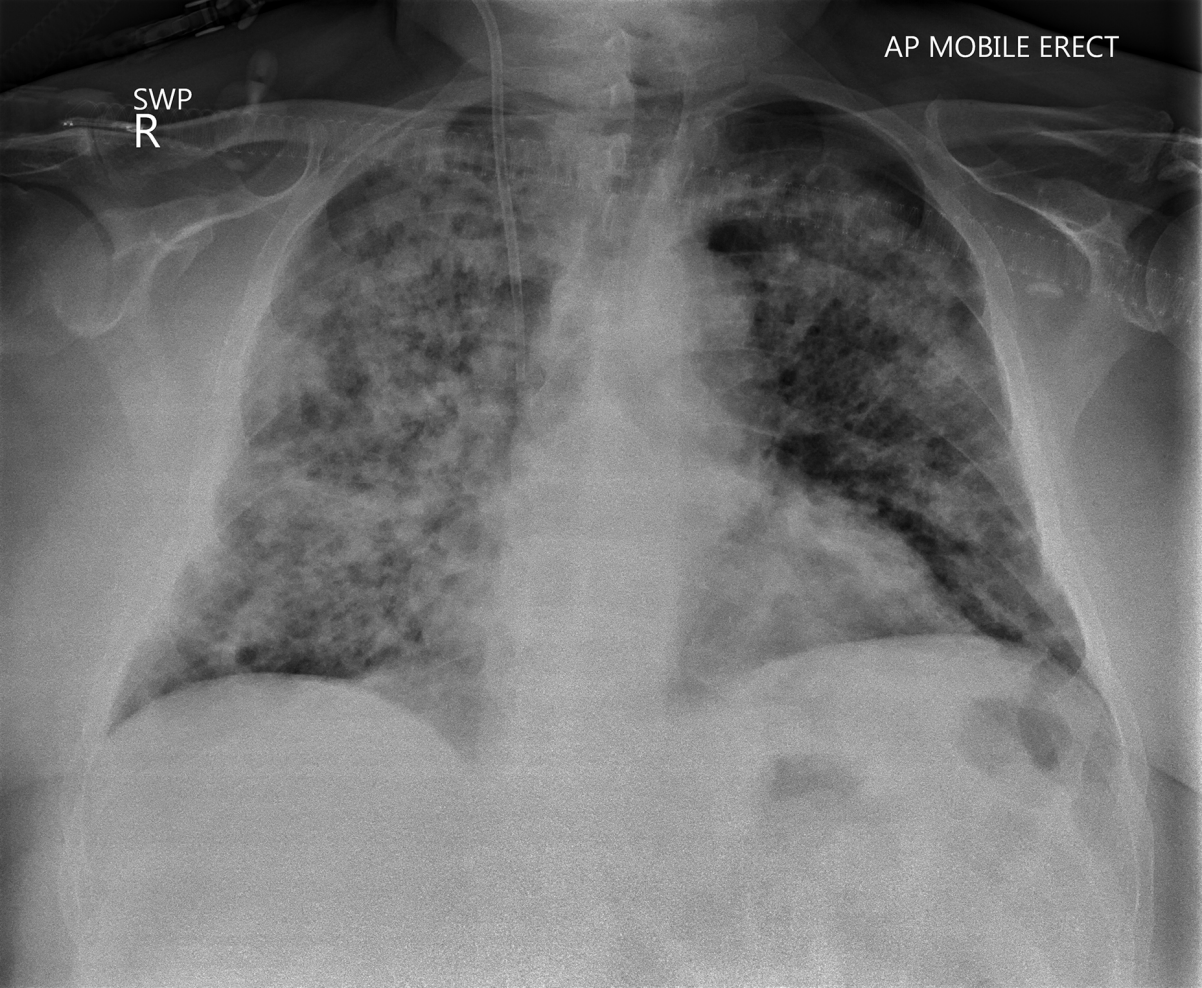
Chest radiograph at day 3 of admission. Note significant bilateral but asymmetrical infiltrates.
